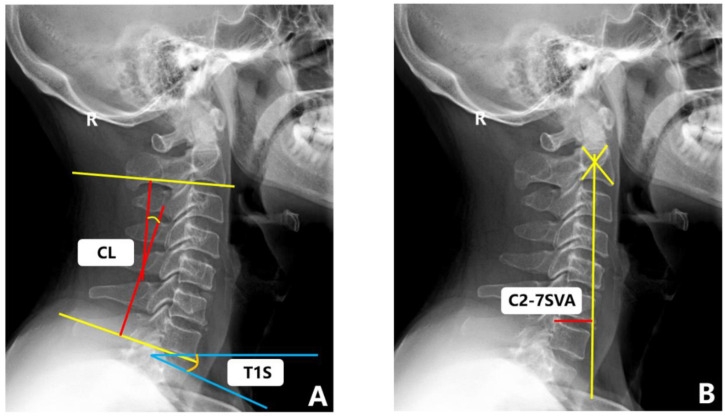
Sagittal radiograph of cervical spine with representative measurements. (A) C2-C7 cobb angle (CL), T1 slope (T1S). (B) C2-7SVA.
